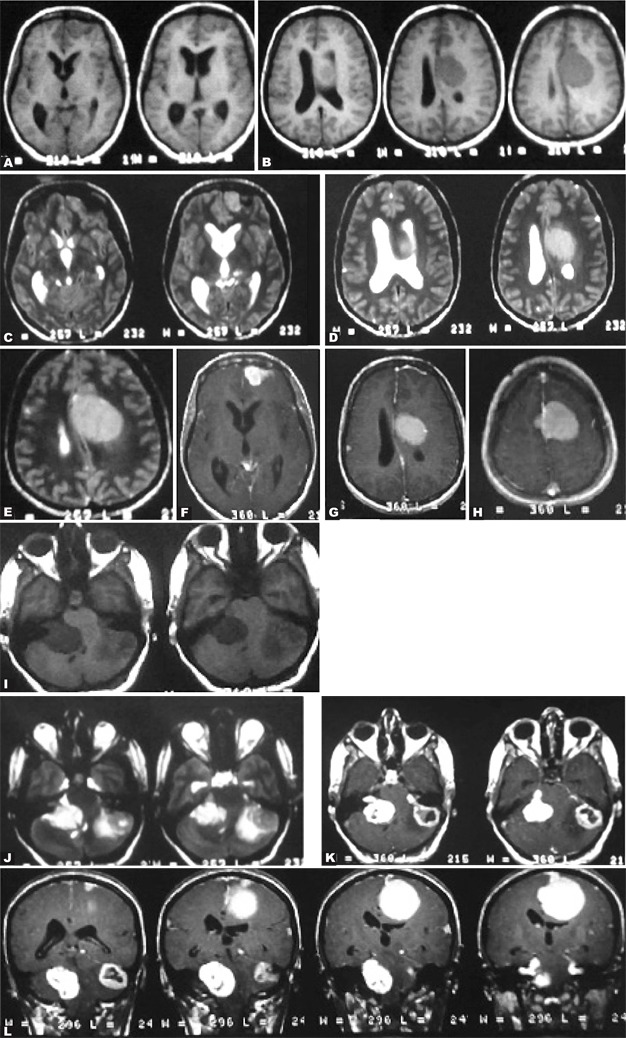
A 24-year-old girl with bouts of headache and sensory-neural hearing loss. A and B. Axial T1-weighted MR images of the brain. C-E. Axial T2-weighted MR images. F-H. Axial T1-weighted MR images with contrast. I. Axial T1-weighted MR images without contrast. J. Axial T2-weighted without contrast MR image of the CP-angles. K and L. Axial and coronal post-contrast T1-weighted MR images.
